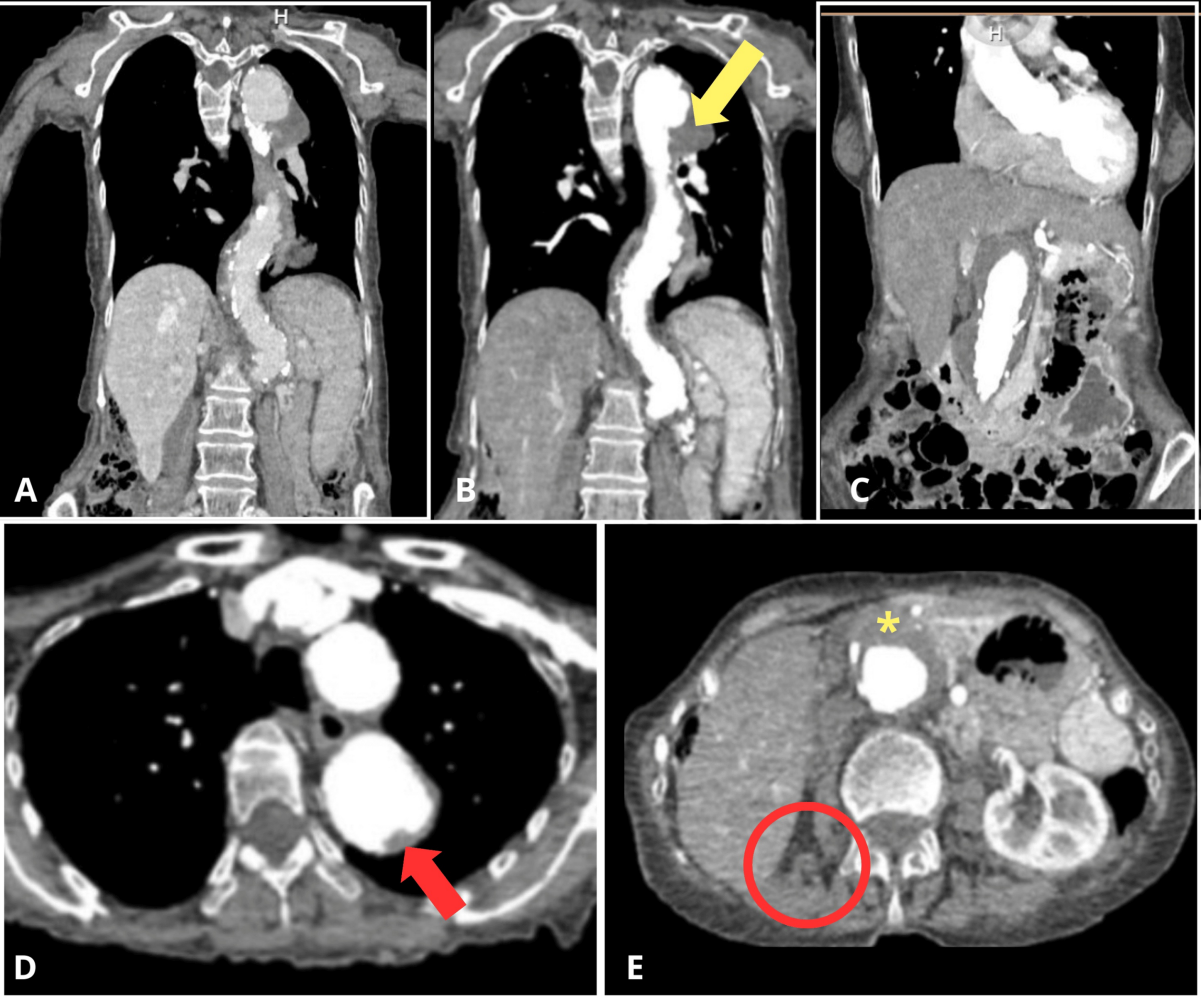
Contrast-enhanced computed tomography A: Coronal CT angiography demonstrates a DeBakey type IIIB aortic dissection, with fusiform dilation of the descending thoracic and abdominal aorta as well as multiple calcifications. B: Coronal contrast-enhanced CT angiography, a hypodense lesion consistent with saccular aneurysmal degeneration is identified at the level of the descending thoracic aorta. The aneurysmal sac contains an intramural thrombus, as evidenced by the heterogeneous density within the aneurysm (yellow arrow). C: Coronal contrast-enhanced CT shows an intramural thrombus in the distal abdominal aorta, extending caudally to near the aortic bifurcation. It appears as a well-defined hypodense area along the posterior wall, without significant luminal narrowing, dissection, or contrast extravasation. D: Axial CT angiography reveals an intramural thrombus (indicated by the red arrow), originating just distal to the origin of the supra-aortic branches. E: Axial CT angiography reveals right renal atrophy (red circle), with no contrast enhancement of the right renal artery or renal parenchyma. An intramural thrombus in the abdominal aorta is indicated by a yellow asterisk.
